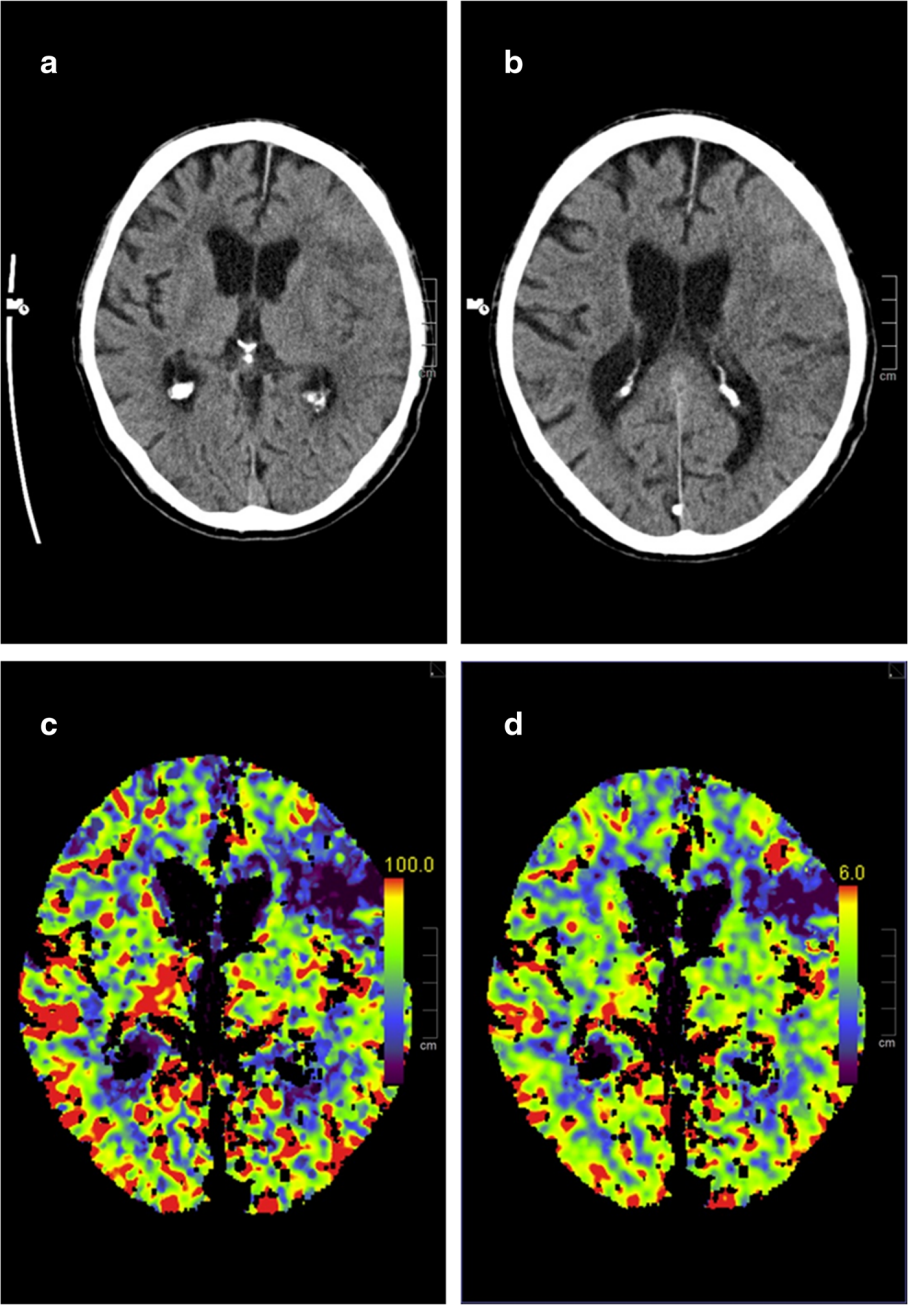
Non-contrast CT at admission showing left-sided MCA-infarction (a, b). CT-perfusion showing reduced perfusion of the left anterior MCA-territory in Cerebral Blood Flow (CBF)- (c) and Cerebral Blood Volume (CBV)-measurements (d)
